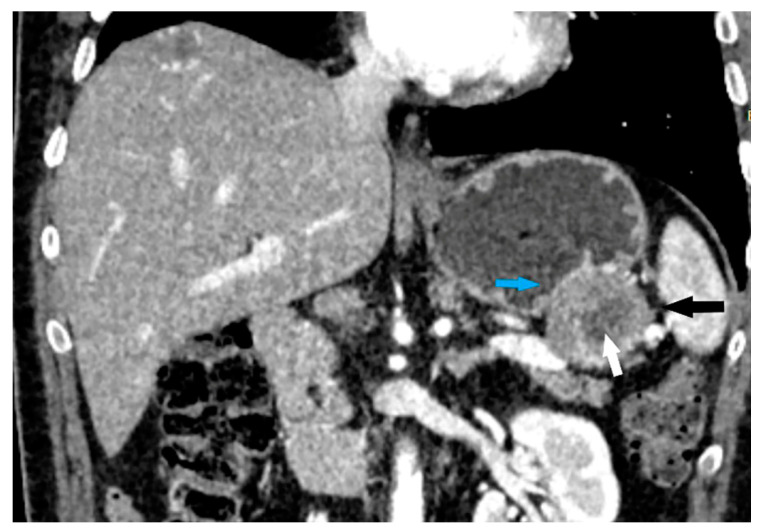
CT scan coronal view clearly depicts oval, clearly demarcated lesion (black arrow) covered by intact mucosa (blue arrow) with partially necrotic structure (white arrow) and weak postcontrast opacification of solid part of the lesion.
